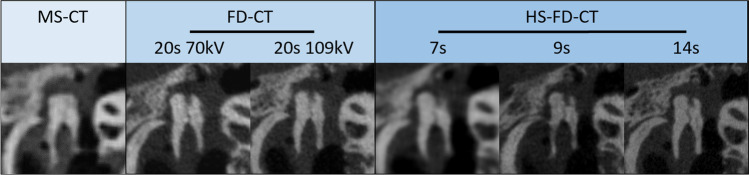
MS-CT, FD-CT, and HS-FD-CT MRPs aligned to the incudomalleolar joint comparing different scanning protocols
